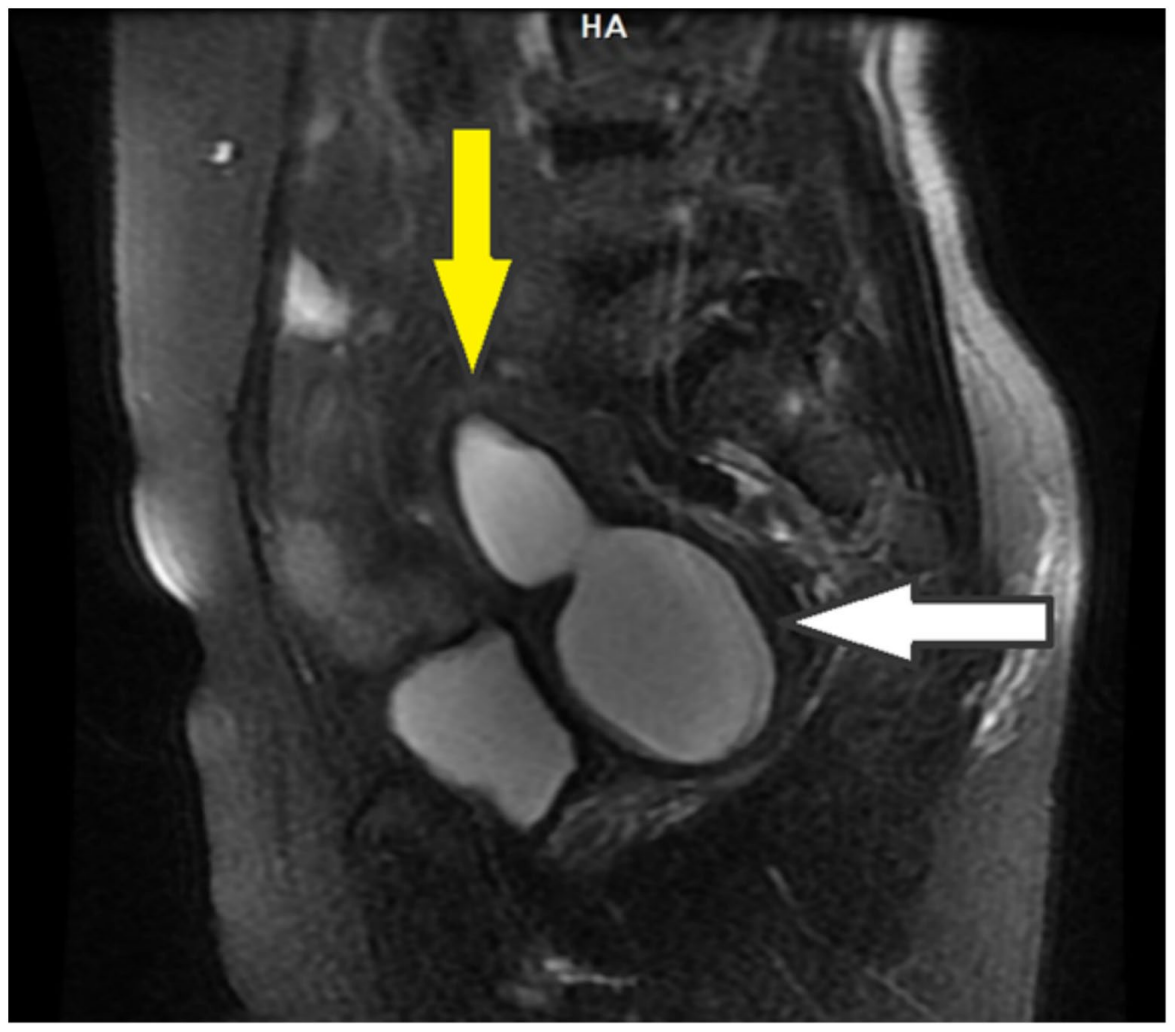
MRI: Sagittal T2-weighted image shows the presence of hematocolpos (white arrow) and hematometra (yellow arrow).
